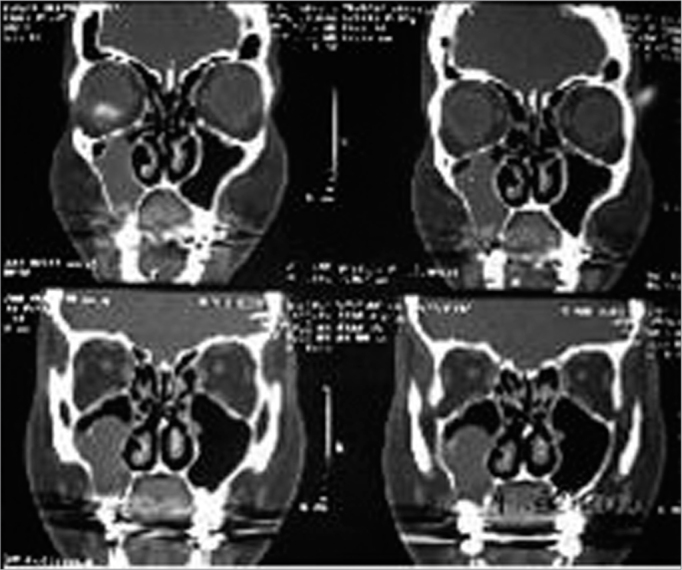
Coronal view CT scan showing a tumor invading the right maxillary sinus.
